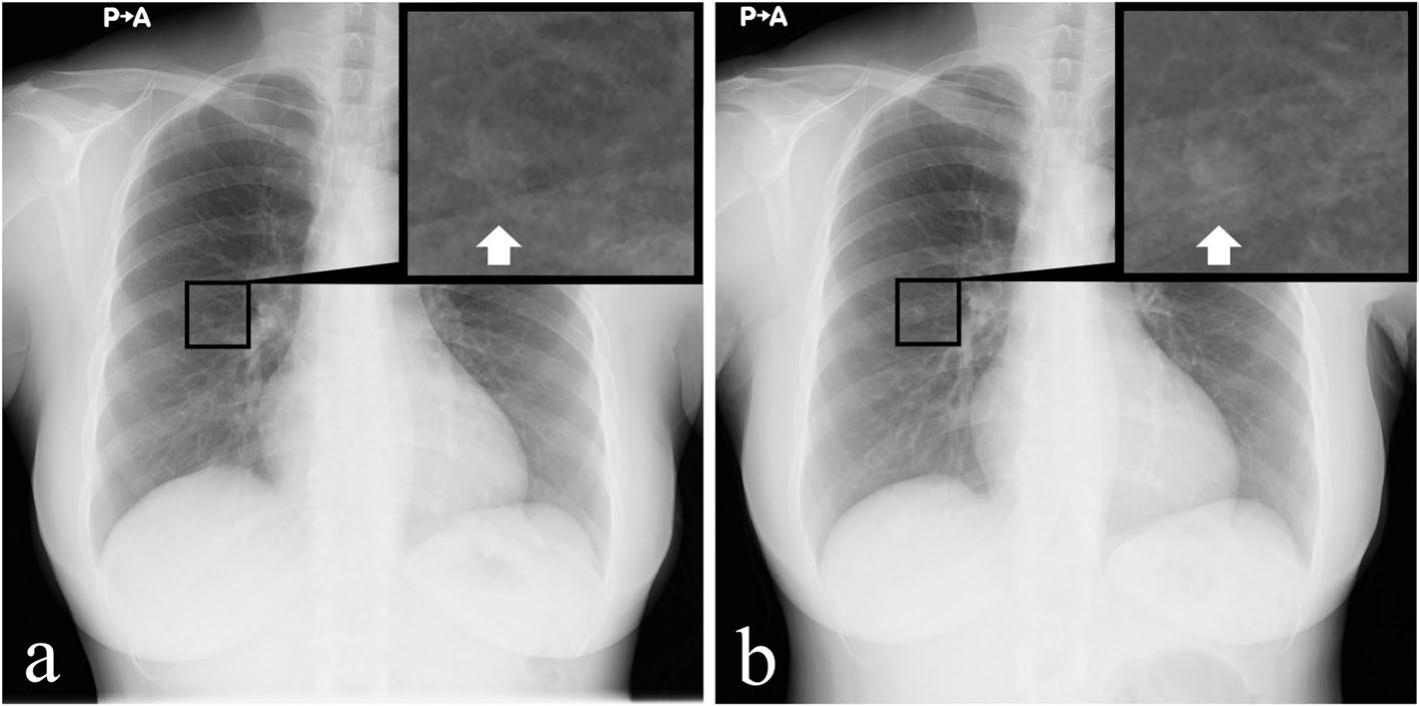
Chest X-ray findings: a A cyst with an intracavitary nodule was seen in the right middle lung field. b One year later, the intracavitary nodule had increased in size (enlarged view, arrowhead)
